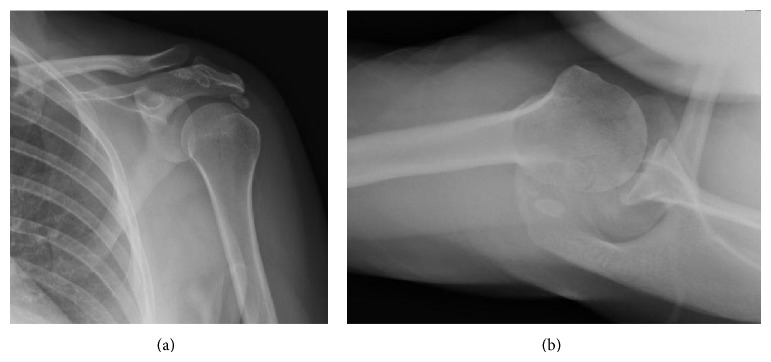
Preoperative (a) anteroposterior and (b) axillary lateral radiographs of the left shoulder showing periarticular calcified nodules and erosion of the AC joint.
